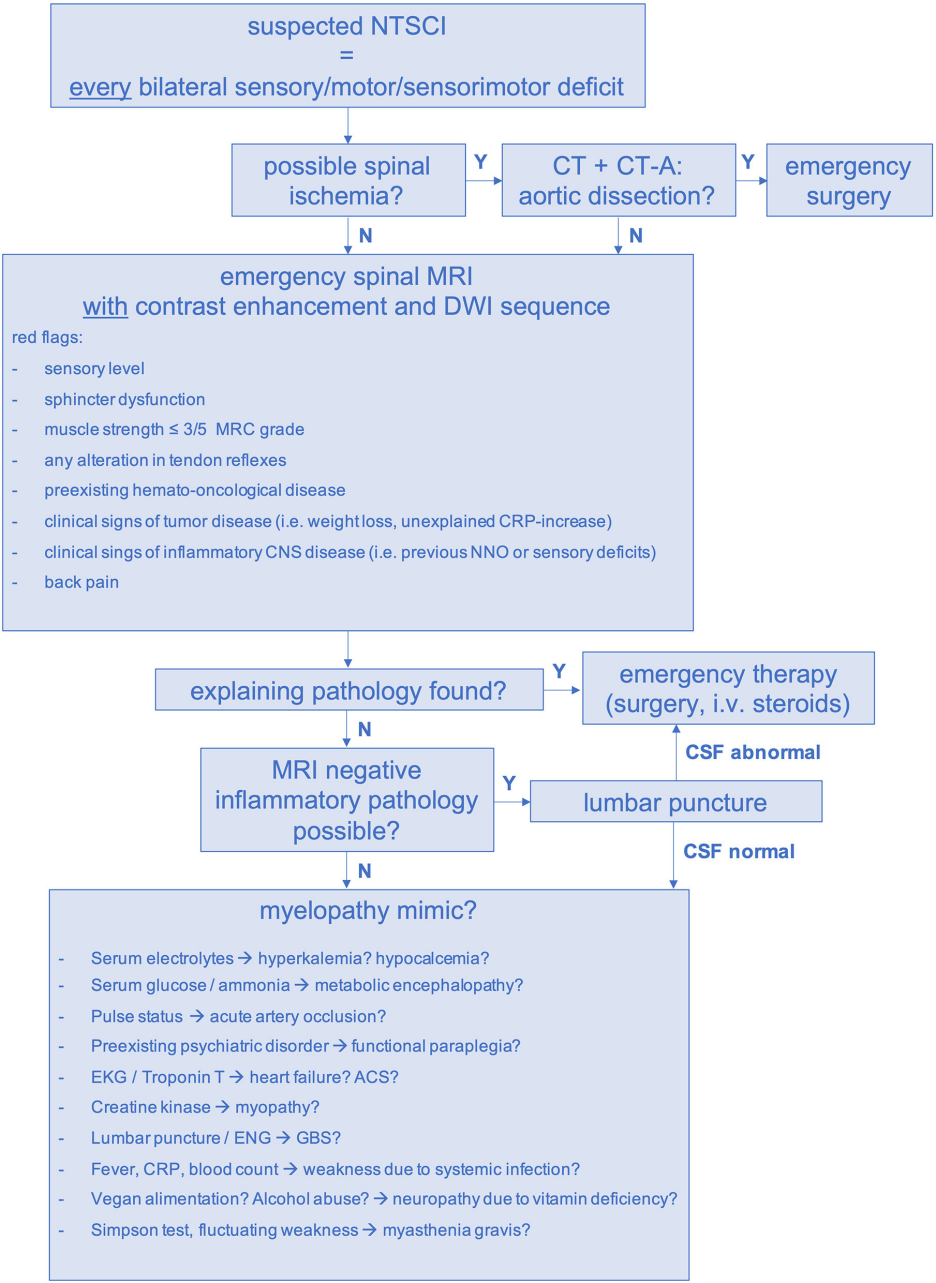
Diagnostic pathway for suspected NTSCI in the emergency setting. ACS, acute coronary syndrome, CNS, central nervous system; CRP, c-reactive protein; CT, computed tomography scan; CT-A, computed tomography angiogram; DWI, diffusion-weighted imaging; ENG, electroneurography, GBS, Guillain-Barré syndrome; MRI, magnetic resonance imaging; N, no; NNO, optic neuritis; NTSCI, non-traumatic spinal cord injury; i.v., intravenous; Y, yes.
